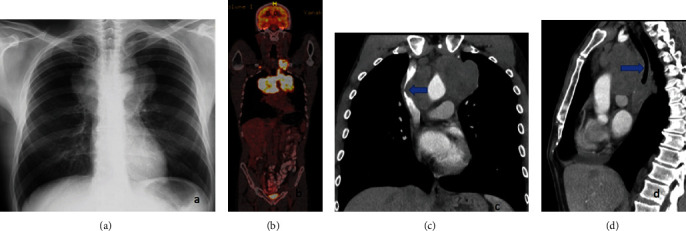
FDG avid lymph nodes in the left supraclavicular region extending to the anterior mediastinum.
